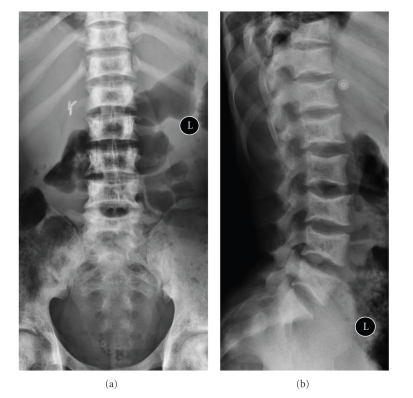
(a) and (b) AP and lateral plain radiographs of the lumbar spine showing sharp end plate depressions due to central end-plate infarction resulting in classic H-shaped vertebrae. On the other hand bone softening results in smooth concavity described as fish mouth vertebra. Also note cholecystectomy clips from previous surgery for pigmented stones and patchy sclerosis of the pelvic bones from medullary infarction.
